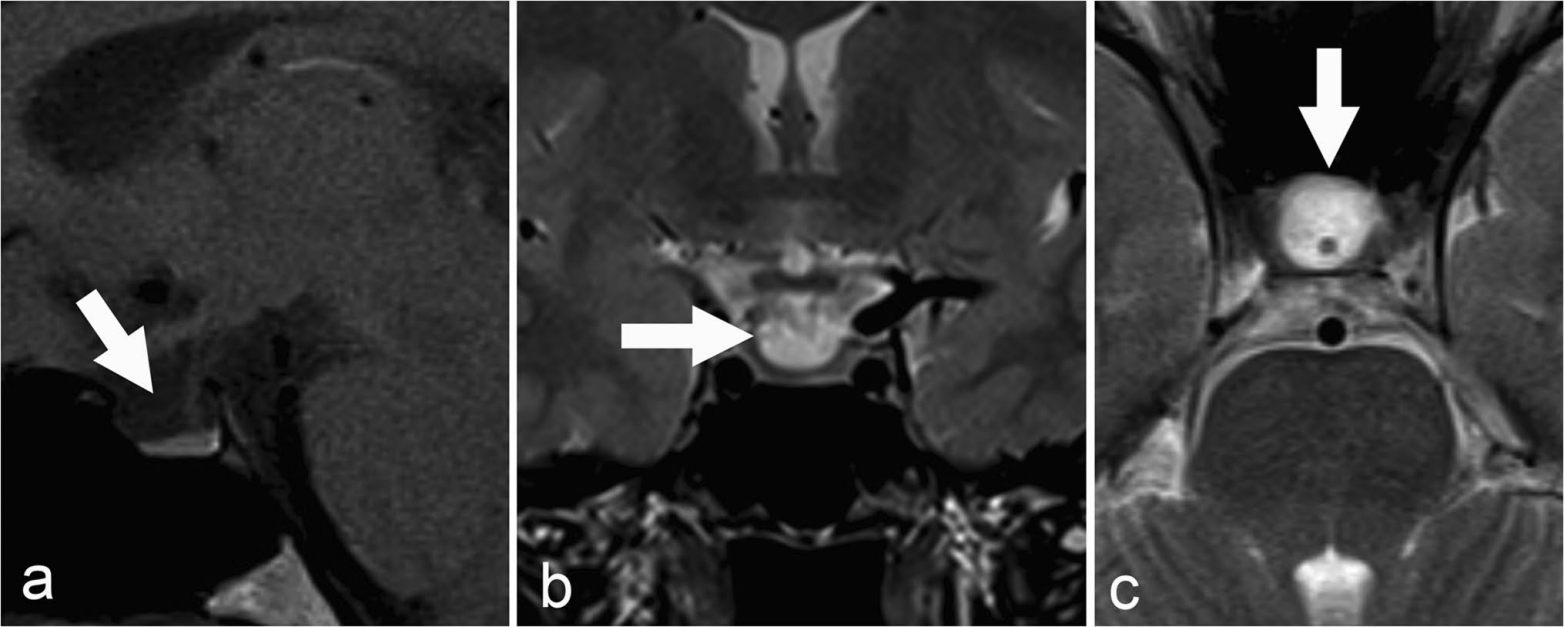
Non-contrast MRI (panels a–c) of the pituitary gland with sagittal (a) T1-weighted as well as coronal (b) and axial (c) T2-weighted dedicated 3-mm thin-sections from case 3. There is subtle expansion of the anterior recess of the sella to thicker with flattening of the superior contour of the adenohypophysis (white arrows in panels a–c).
